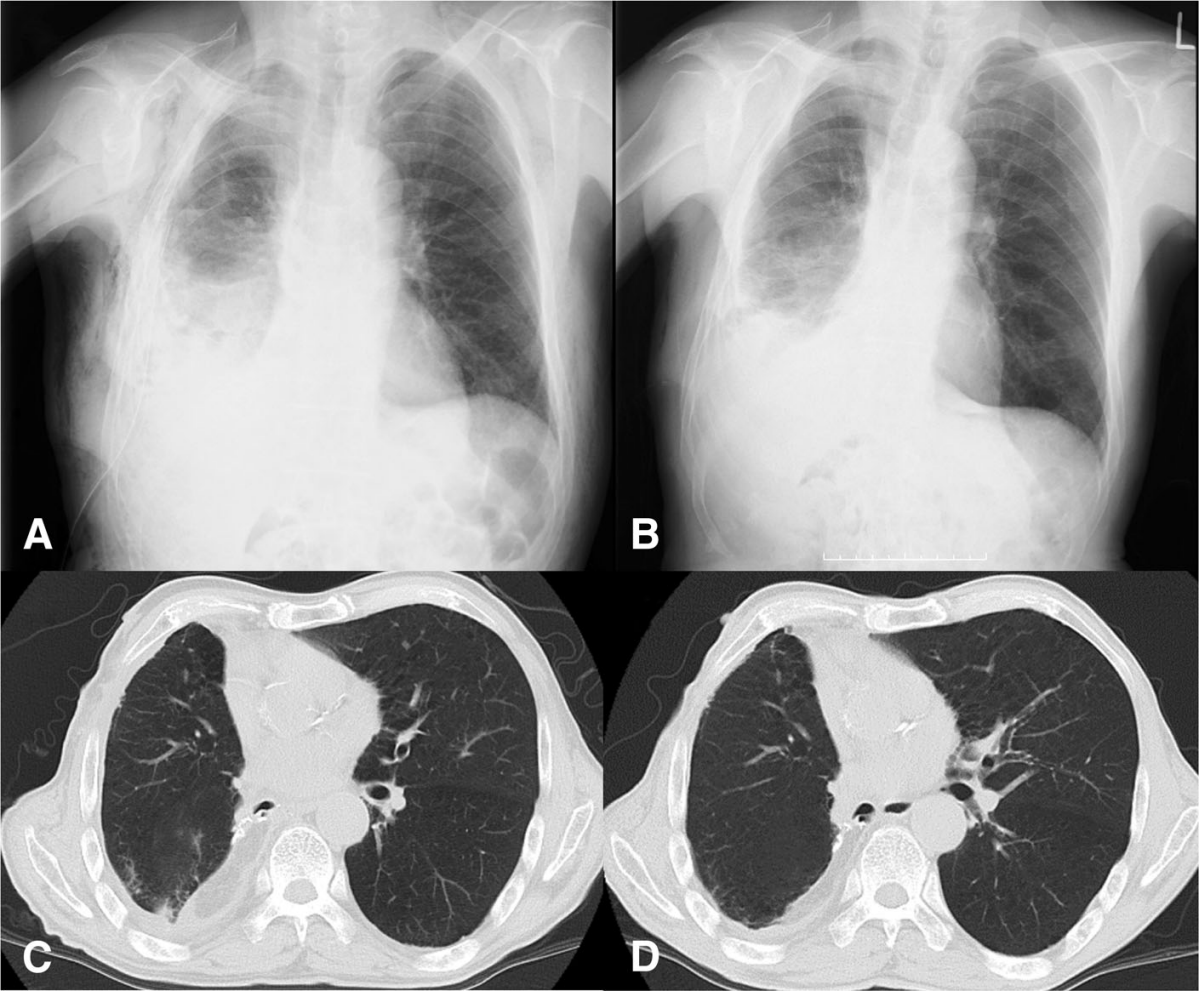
Findings after reoperation. After re-operation, the chest X-ray images on POD 2 (a) and POD 14 (b), as well as the chest CT images at 1 month (c) and at 3 months (d), are shown. CT computed tomography, POD postoperative day
